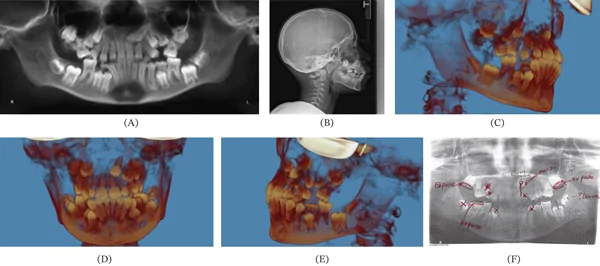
(A) Case #1 preliminary panoramic radiograph. (B) Case #1 preliminary lateral cephalogram. (C–E) Case #1 3D dentoalveolar reconstruction to determine tooth position. (F) Case #1 handwritten planning on panoramic radiograph.
